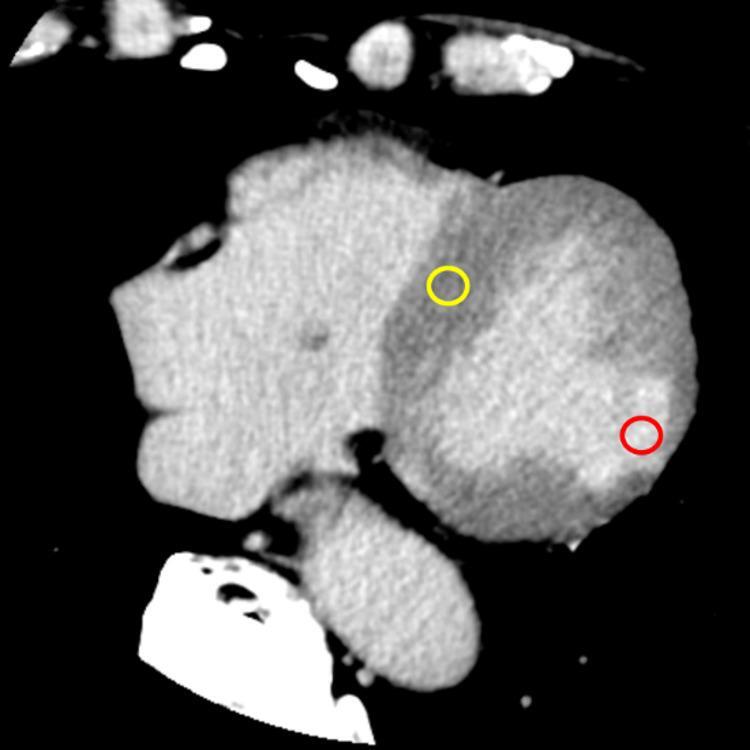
Representative axial image from myocardial delayed enhancement CT showing placement of circular regions of interest (ROIs) for quantitative analysis. The yellow circle indicates an ROI placed in the remote myocardium, while the red circle marks an ROI placed within the hyperenhanced myocardium
